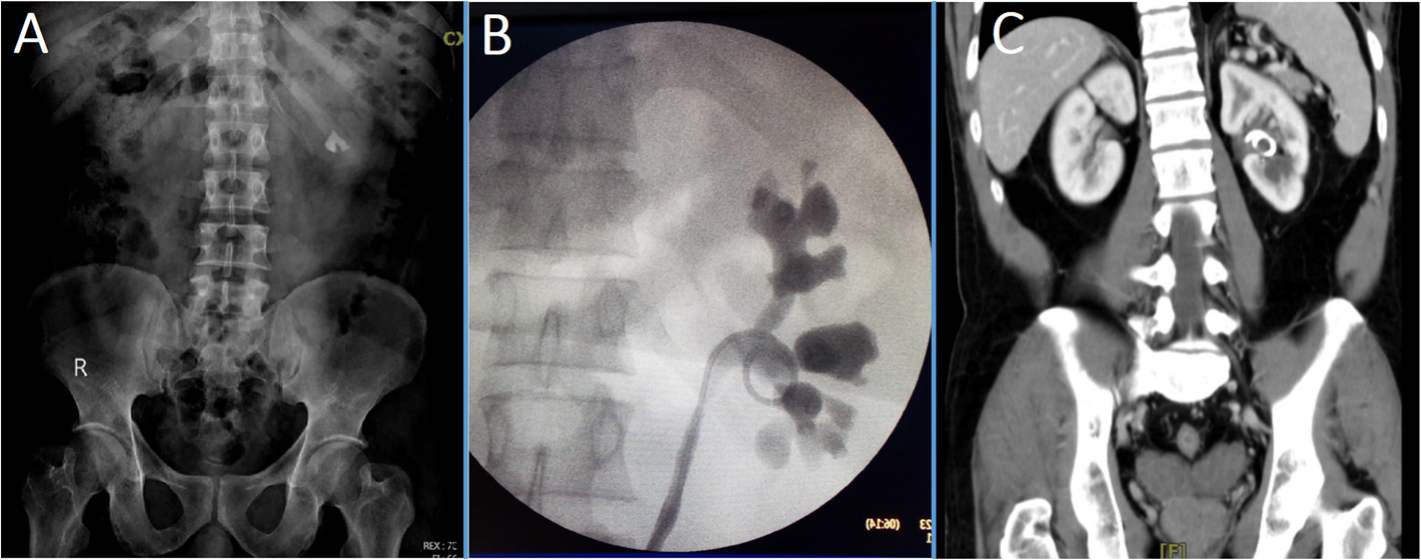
Imaging of the first case. a, Pre-operative plain x-ray with partial staghorn calculus; b, Retrograde pyelography showing the loop of the JJ stent outside the collecting system and the narrow renal pelvis; c, CT scan showing the upper coil of the JJ stent
